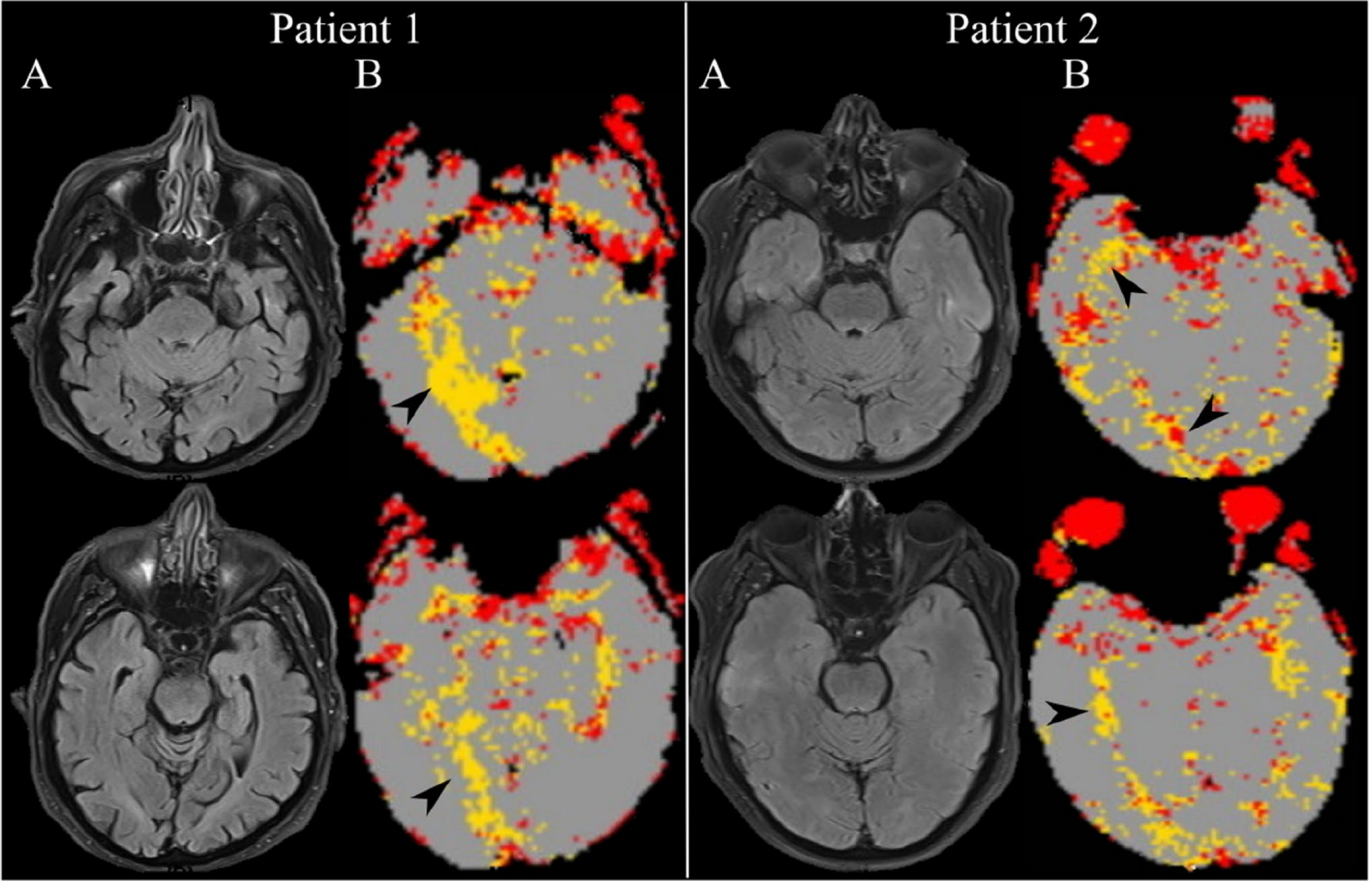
Patient 1 (left) and Patient 2 (right): A fluid attenuated inversion recovery (FLAIR) and B the corresponding perfusion-weighted imaging (PWI) maps (Olea Sphere®, Olea Medical) showing hypoperfusion in the right mesial occipito-temporal cortex in both patients (black arrows)
